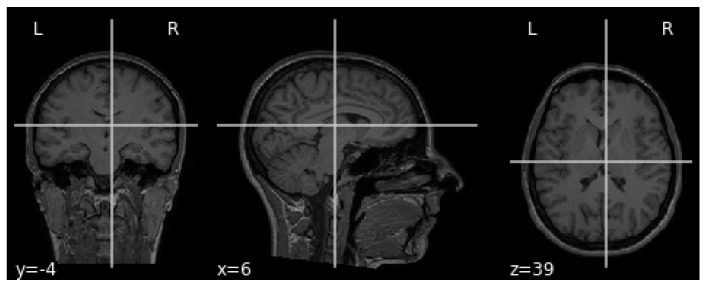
Axial MRI sample of a normal person with healthy brain.
